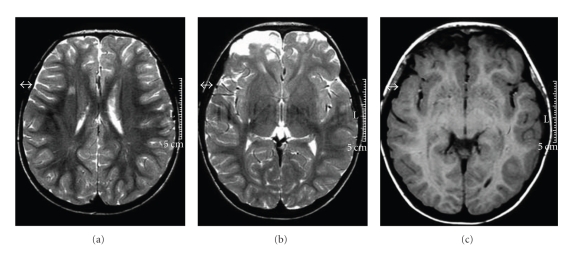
A control MRI was performed 2 years after trauma: FLAIR section (a) showed stable white matter lesions (right fronto-parietal and left posterior-parietal), T2-weighed (b) and T1-axial (c) sections showed worsening in bi-frontal atrophy.
